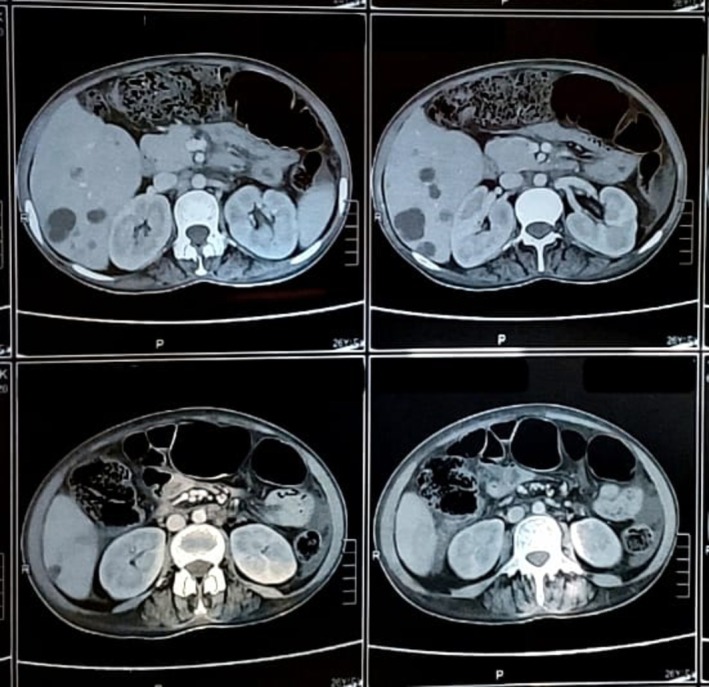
Abdominal CT scan showing nonhomogeneous liver texture and mild abdominopelvic ascites.
